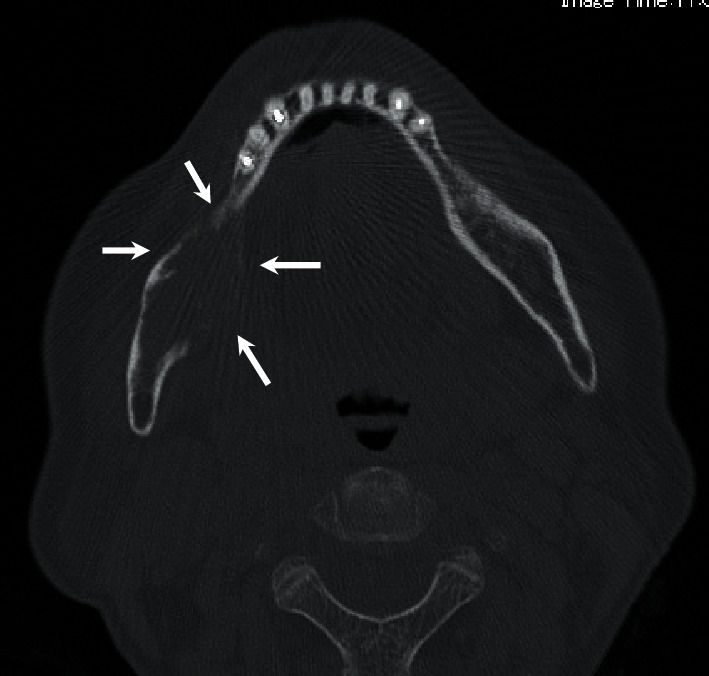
CT image. Axial view. CT depicts thin cortical bone and slightly partially destroyed lingual bone.
